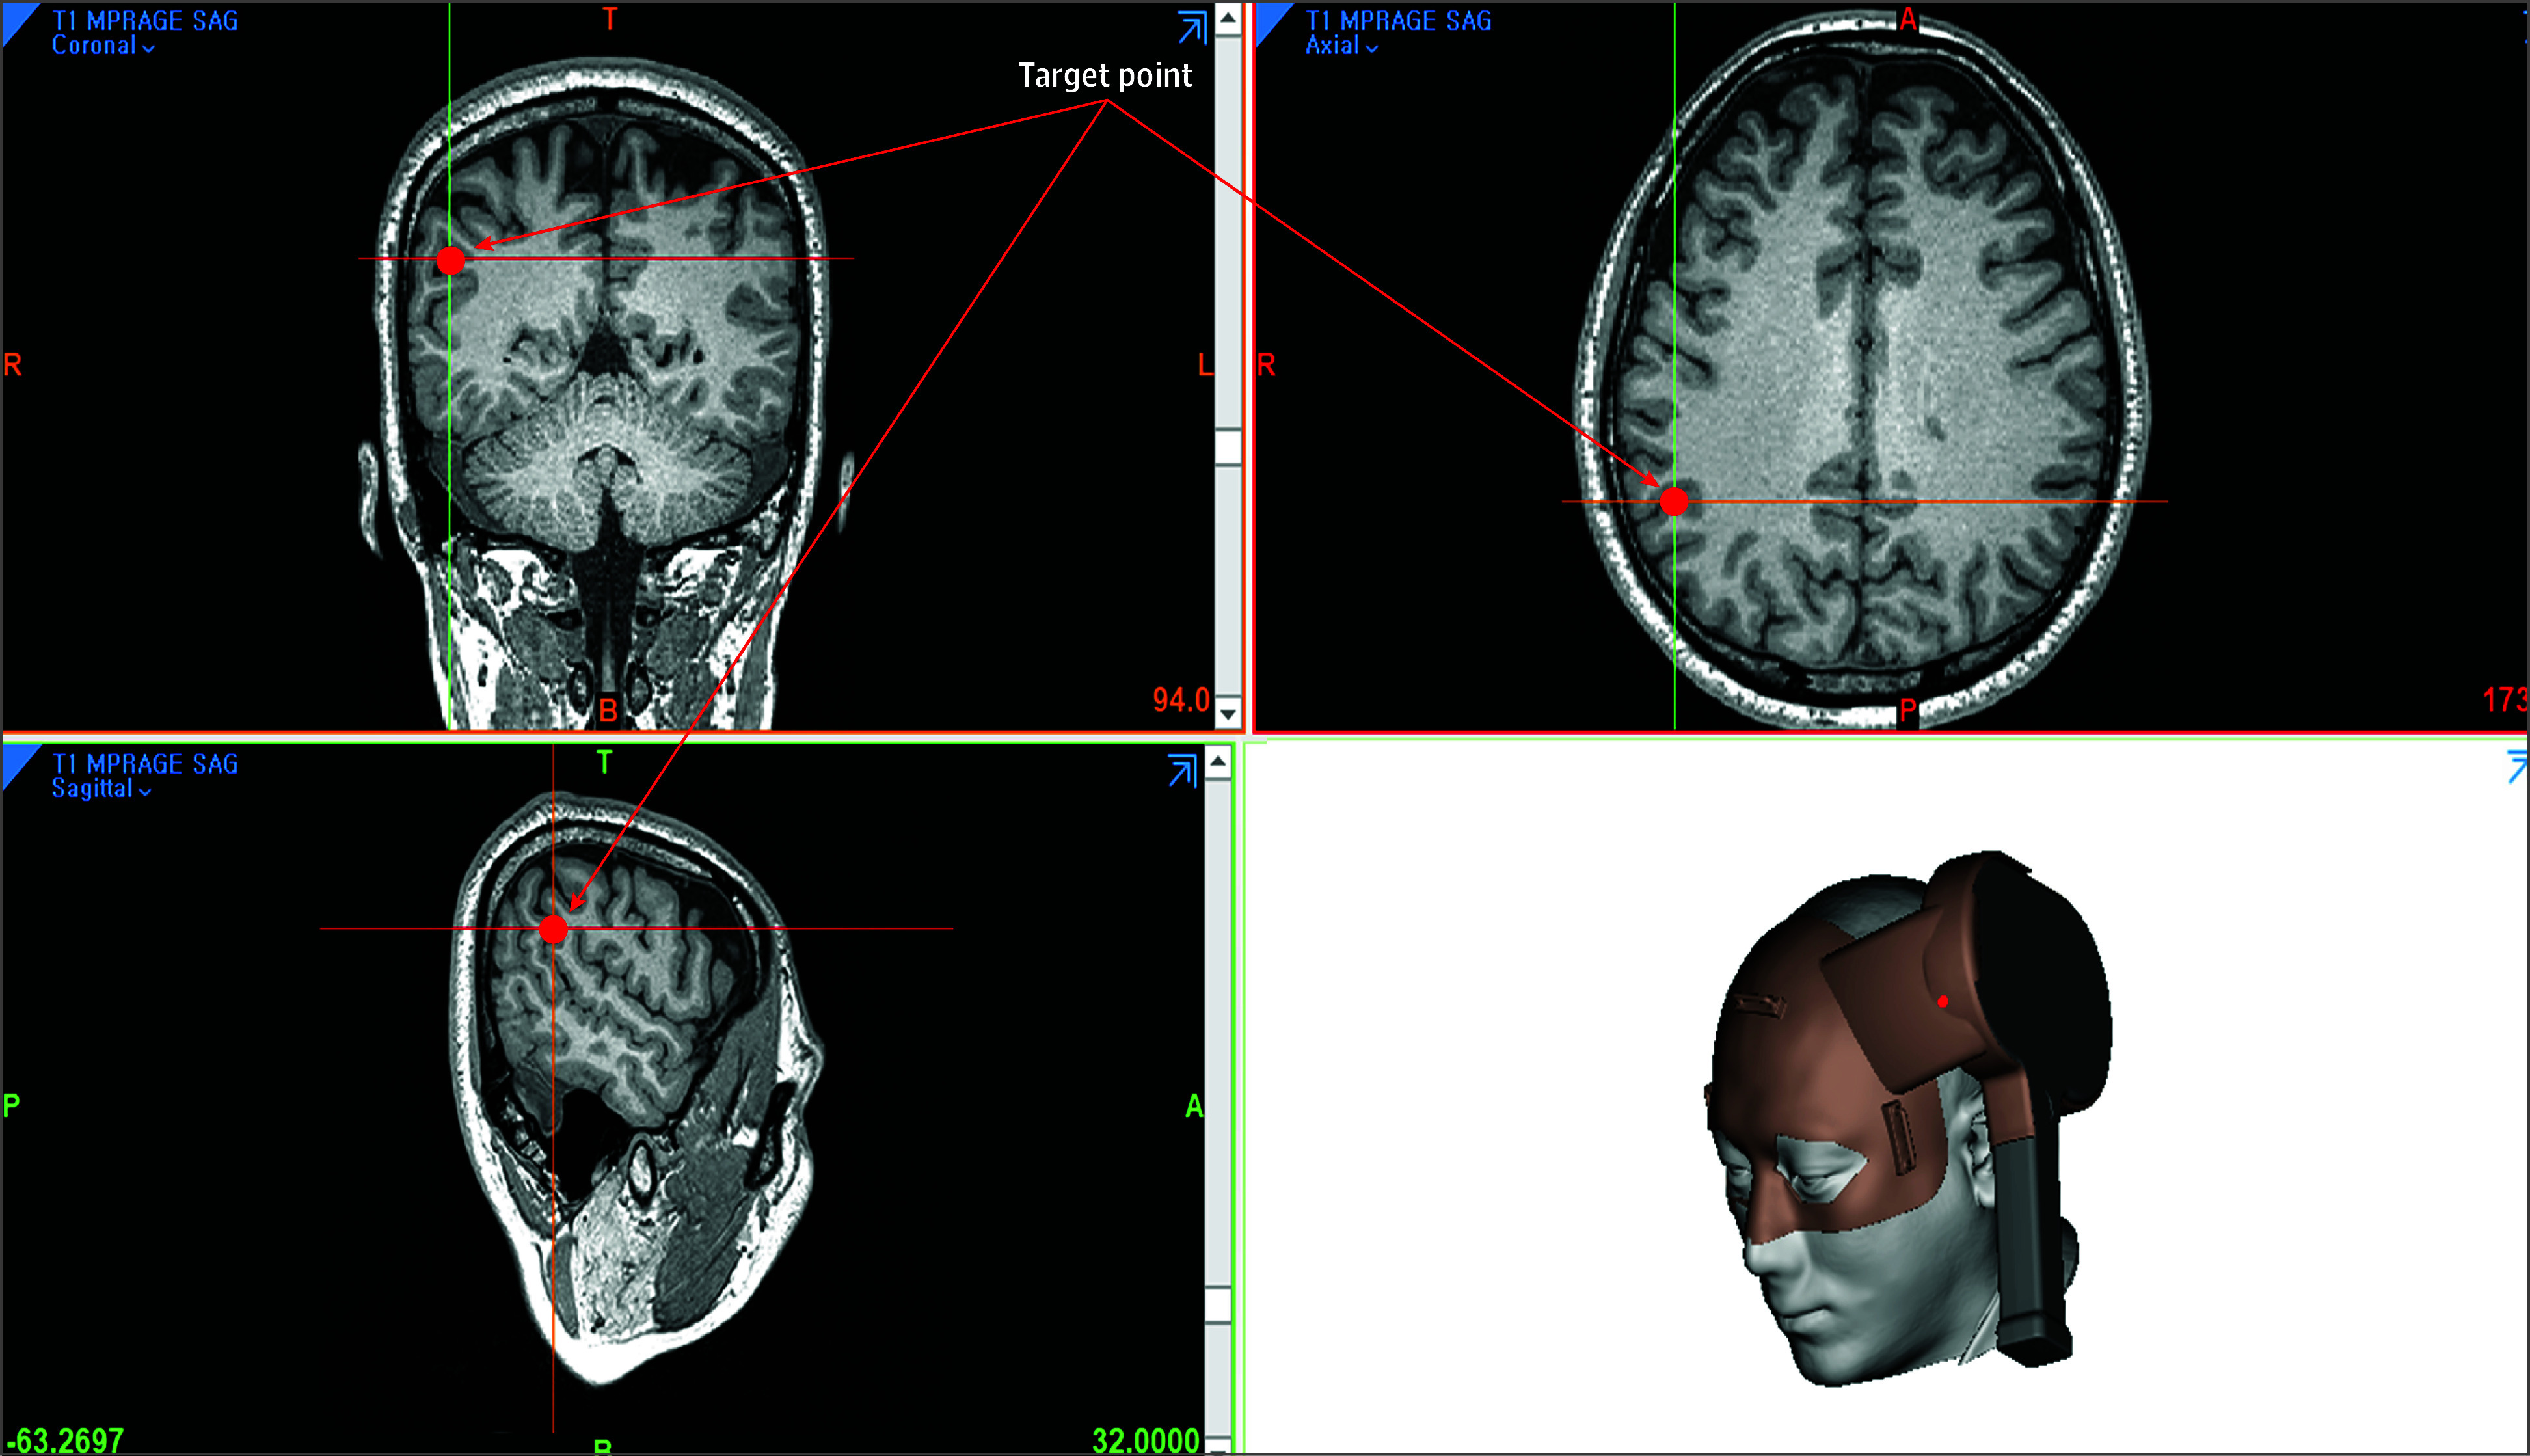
Selection of Target Site and Production of 3-Dimensional (3D) Printed Frame After a target site was determined on the individual T1 structural magnetic resonance image, a personalized 3D-printed frame was produced to fix the transcranial magnetic stimulation coil on the location of the stimulation target.
